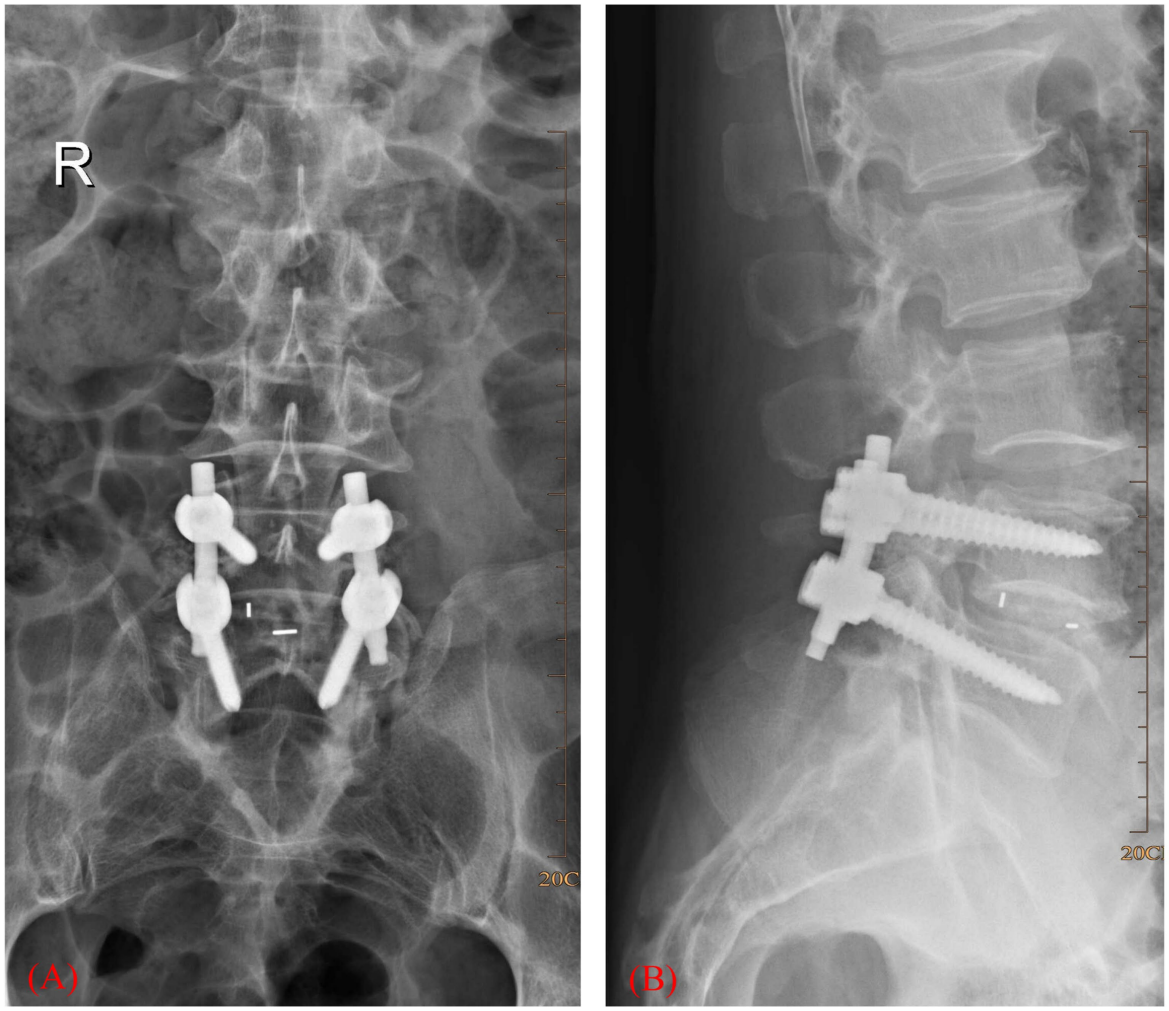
X-ray image showing lumbar interbody fusion surgery. (A) Coronary plane. (B) Sagittal plane.
